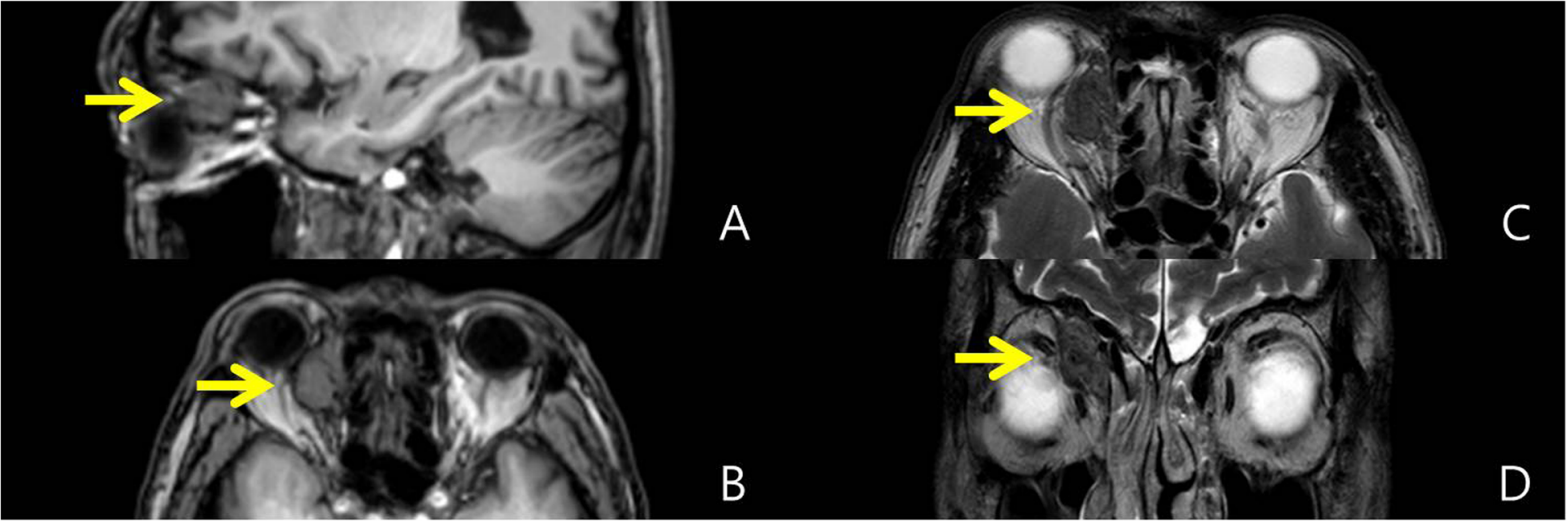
a T1-sagittal and b T1-axial, c T2-axial, and d T2-coronal magnetic resonance images reveal a solid mass (2.4 × 1.5 × 2.0 cm) at the superomedial aspect of the right orbit with T1 and T2 iso-signal intensity. There was no interval change in the mass compared with a previous CT
